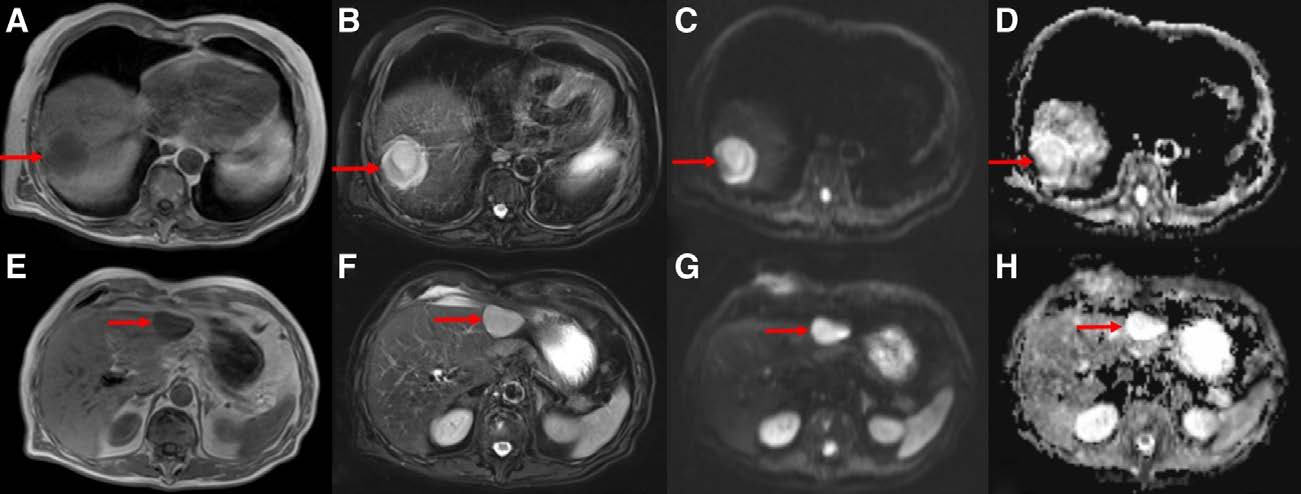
Liver MRI without contrast showing lesions in both the right and left liver lobes. T1WI images (A/E), T2WI image (B/F), DWI image (C/G), ADC image (D/H). ADC = apparent diffusion coefficient, DWI = diffusion-weighted imaging, MRI = magnetic resonance imaging, T1WI = T1-weighted imaging, T2WI = T2-weighted imaging.
